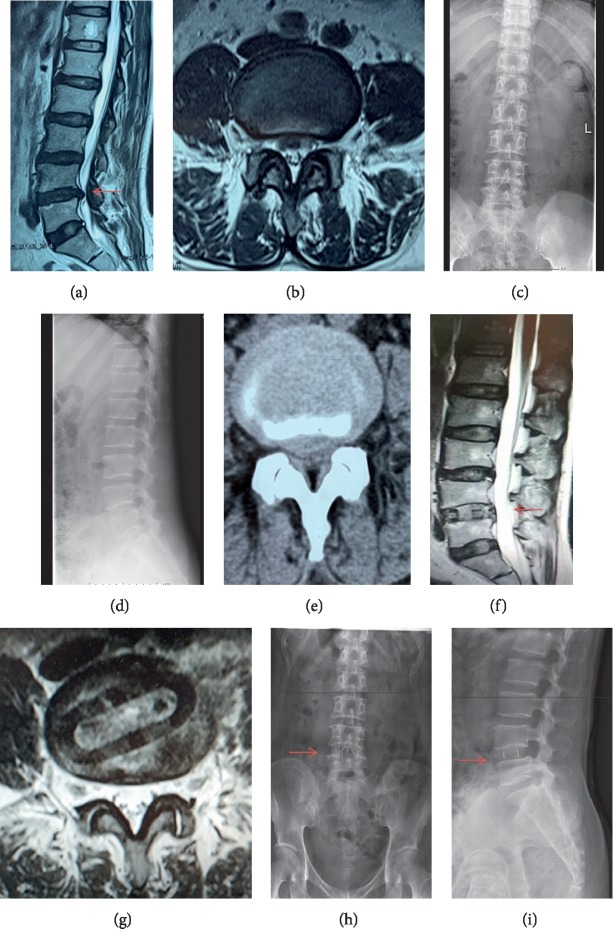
A representative case of OLIF surgery with radiographs and MRI scan: (a, b) preoperative MRI scan, (c, d) preoperative X-ray radiographs, (e) preoperative axial CT scan, (f, g) postoperative MRI scan, and (h, i) postoperative X-ray radiographs.
